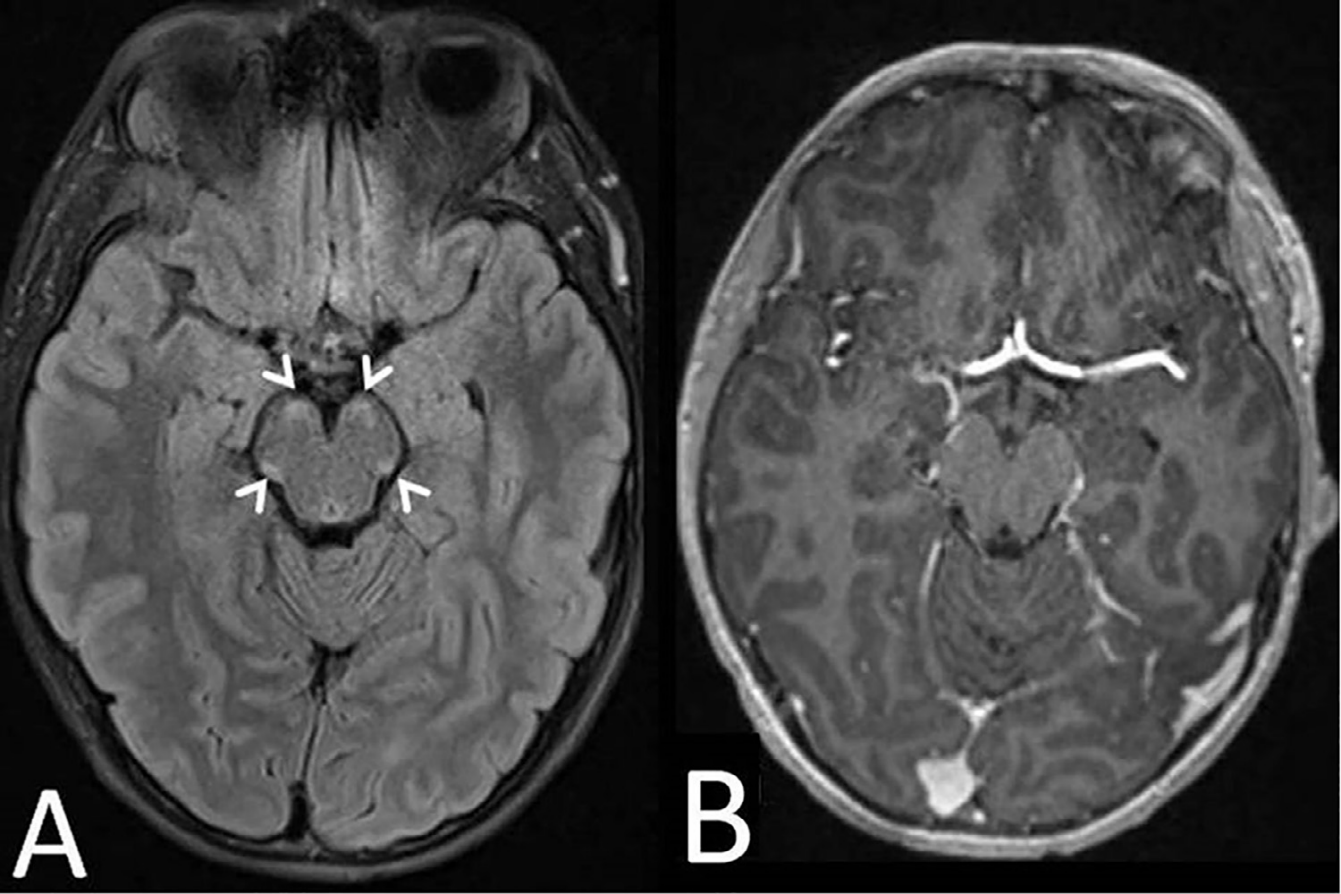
(A) Axial MRI brain without contrast on 9-20-19 with T2 fluid attenuated inversion recovery (FLAIR) with arrowheads showing hyperintense lesions of the cerebral peduncles. (B) Axial MRI brain with gadolinium contrast on 9-20-19 with T1 sequence at the level of the cerebral peduncles showing no contrast enhancement of these lesions.
